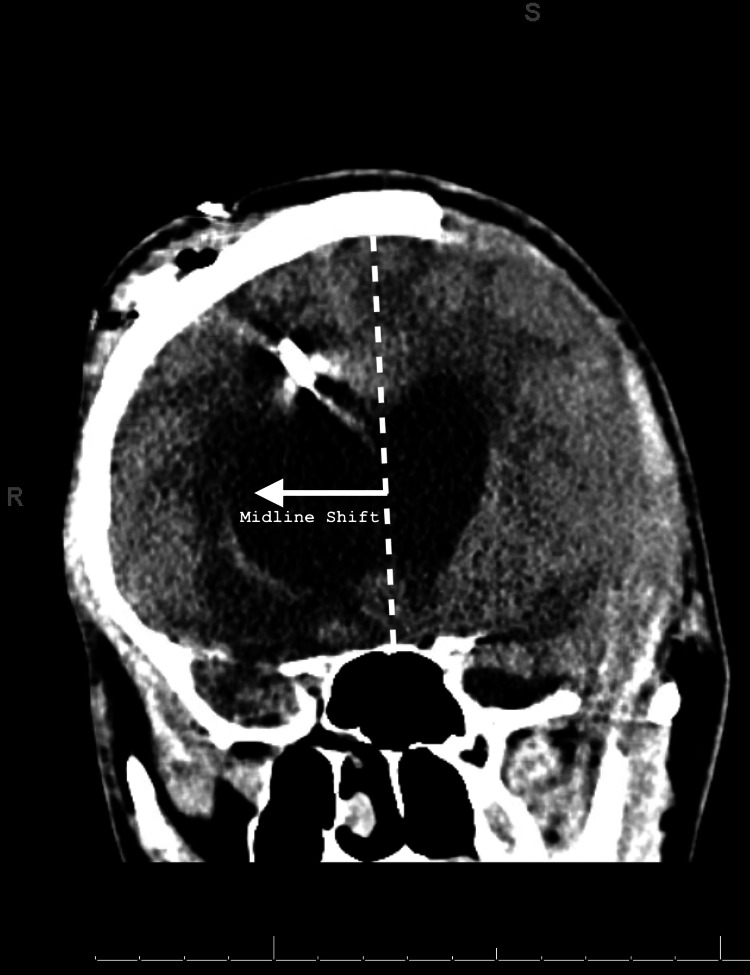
Coronal CT brain demonstrating progressive hydrocephalus with worsening ventricular dilation, diffuse transependymal edema, and marked rightward subfalcine herniation measuring approximately 1.3 cm with midline shift (white arrow), concerning for elevated intracranial pressure secondary to shunt dysfunction. CT: computed tomography
